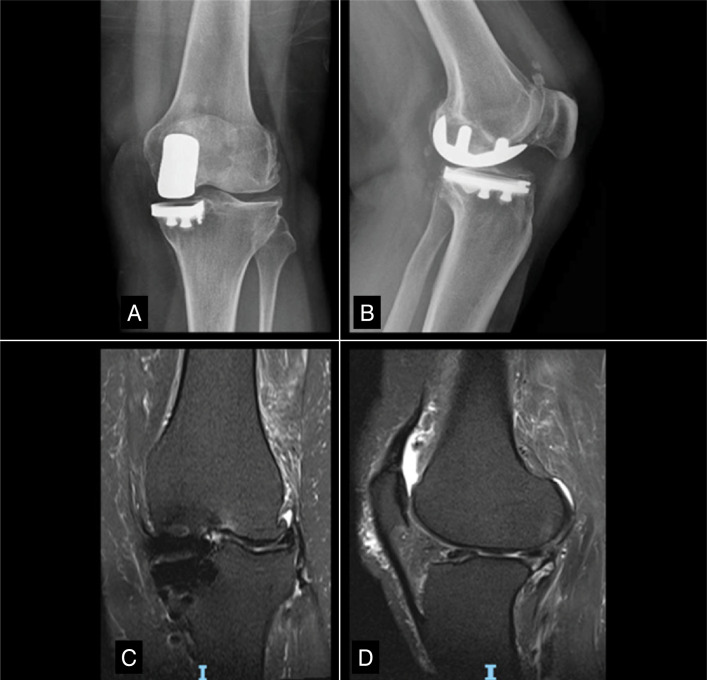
Preoperative X-rays in AP and lateral views (A, B) and coronal and sagittal sections (C, D) from MRI
